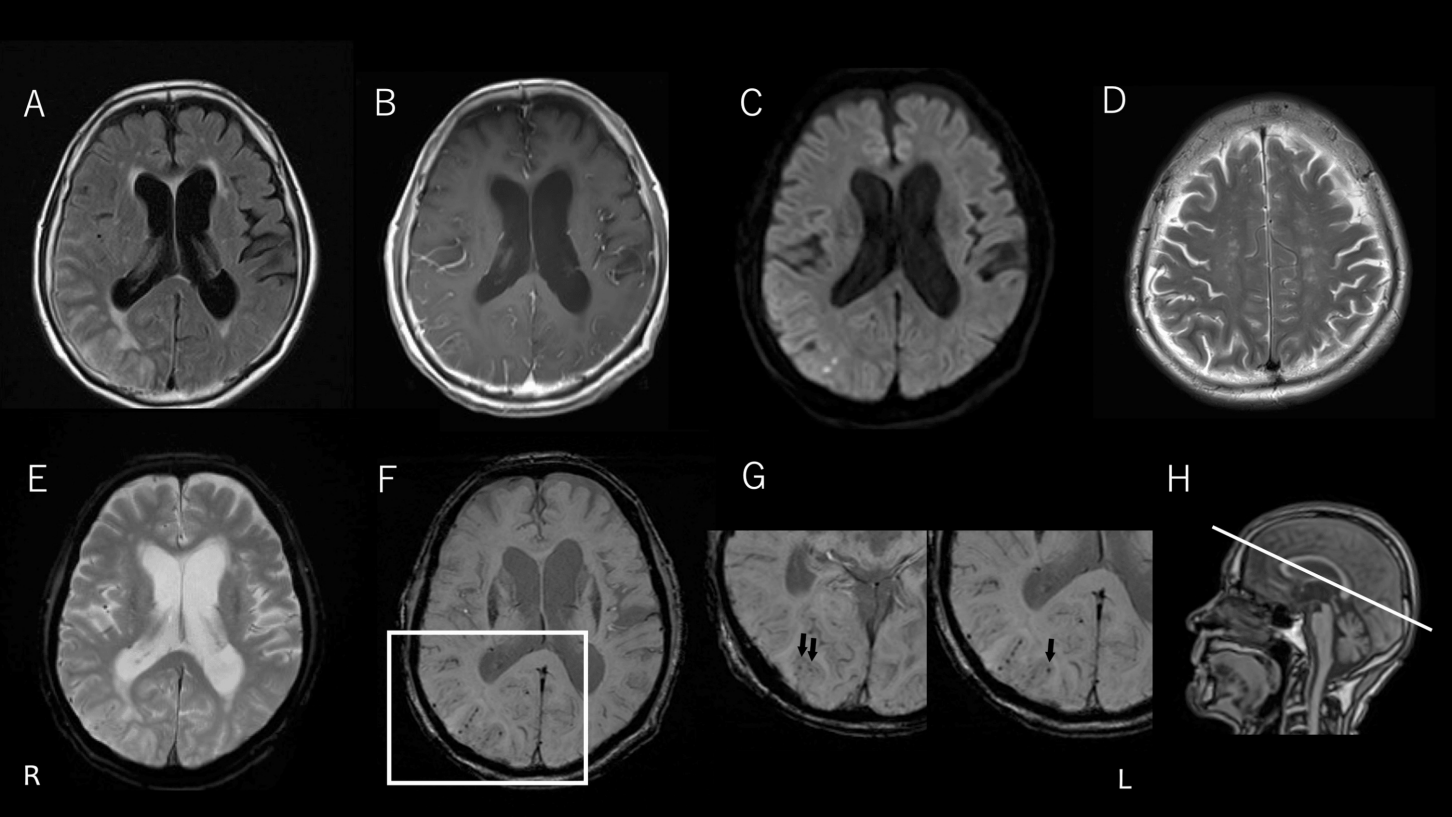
MRI findings at initial presentation. Fluid-attenuated inversion recovery imaging shows multifocal white matter hyperintensities, predominantly in the right occipital lobe (A). Contrast-enhanced T1-weighted imaging reveals leptomeningeal enhancement (B). Diffusion-weighted imaging demonstrates multiple small cortical and subcortical infarcts (C). T2-weighted imaging shows perivascular space dilation in the centrum semiovale (D). No cerebral microbleeds (CMBs) were seen on T2*, although cortical superficial siderosis was noted (E). A small number of CMBs were detectable on thin-slice susceptibility-weighted imaging (F, G).  A sagittal localizer image demonstrated that the T2* and susceptibility-weighted imaging (SWI) sequences were obtained at the same anatomical levels (H).
